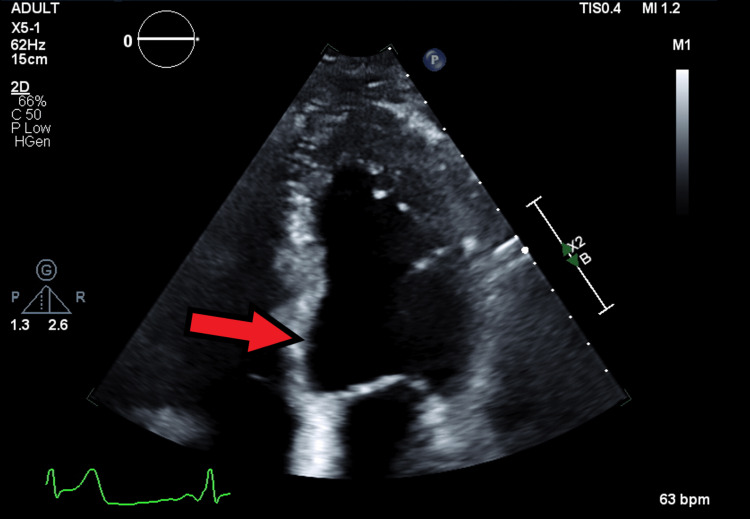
Still of post-procedure echocardiogram; EF of 55%, normal left ventricle size and thickness, and hypokinesis of the basal-mid inferior and inferoseptal wall. EF, ejection fraction.
